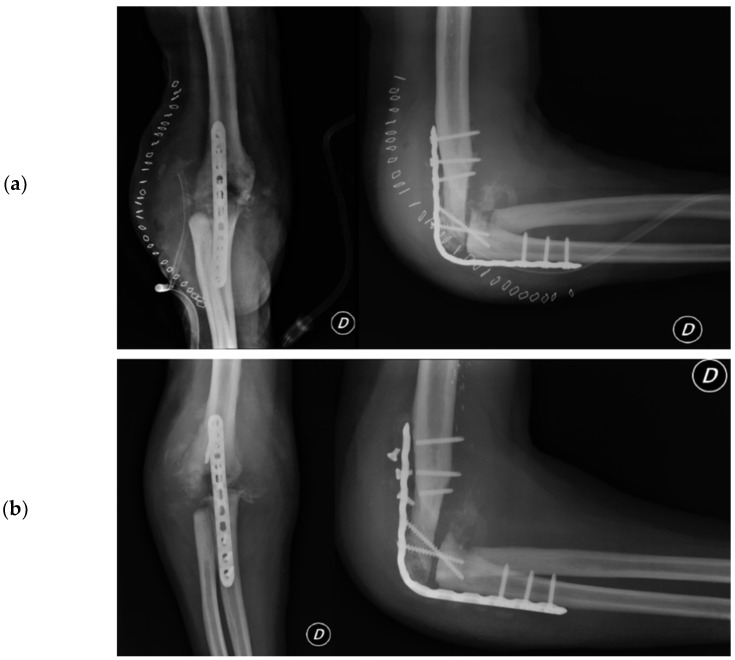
Post-arthrodesis X-ray anteroposterior and lateral views of the right elbow. (a) Post operative images showing the plate in site. (b) Thirty days after images with mobilization of the synthesis with broken screws.
